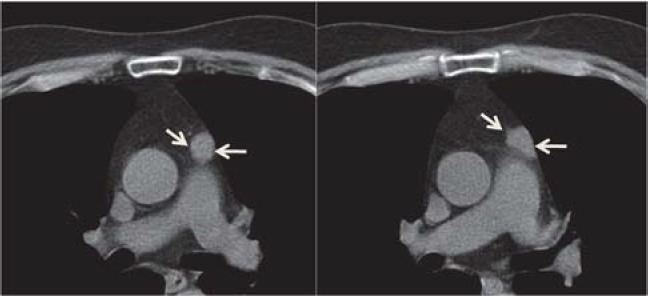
42-y/o male with myasthenia gravis; CT scan shows tumoral nodule in the anterior medistinum (arrows); pathologic diagnosis - type AB thymoma
